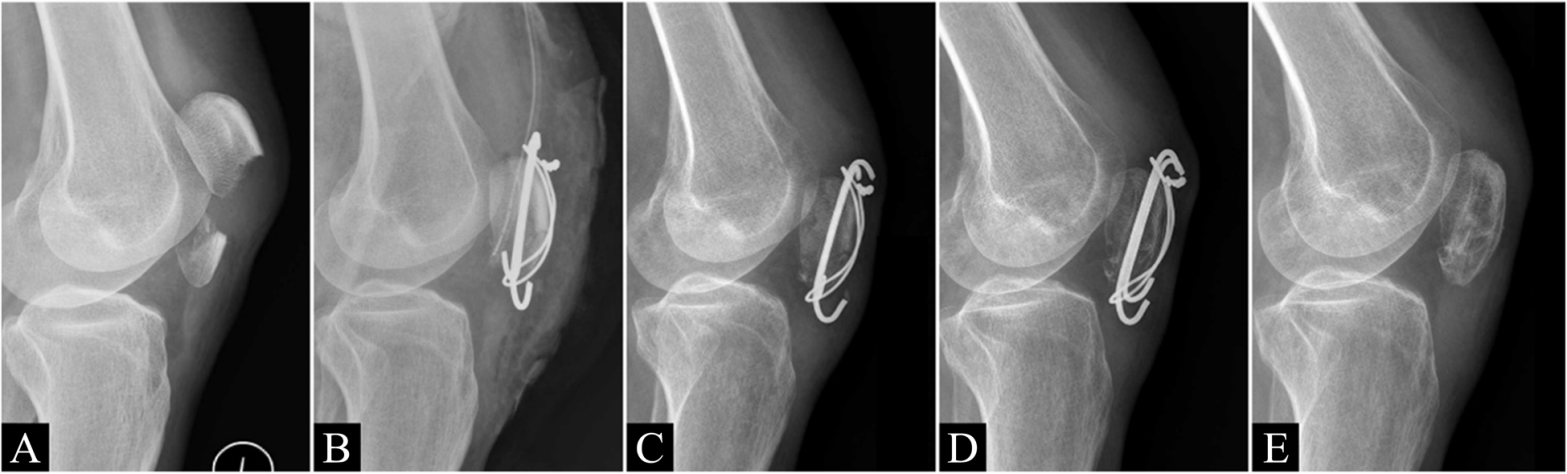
A women aged 53 years underwent tension band wire fixation for left patella lower pole fracture. Knee radiographs A preoperation, B immediately after surgery, C 3 months postoperation, D 12 months postoperation, and E after the removal of implants. The initial postoperative IS ratio was acceptable, but progressive patella baja was noted, even after the removal of implants
